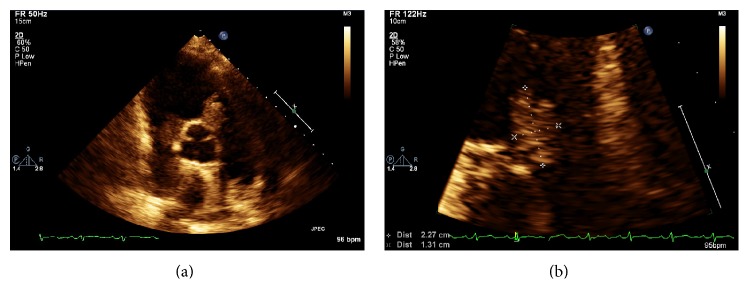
Transthoracic echocardiogram parasternal short axis showing a 2.2 × 1.31 cm echodensity on the pulmonic valve.
